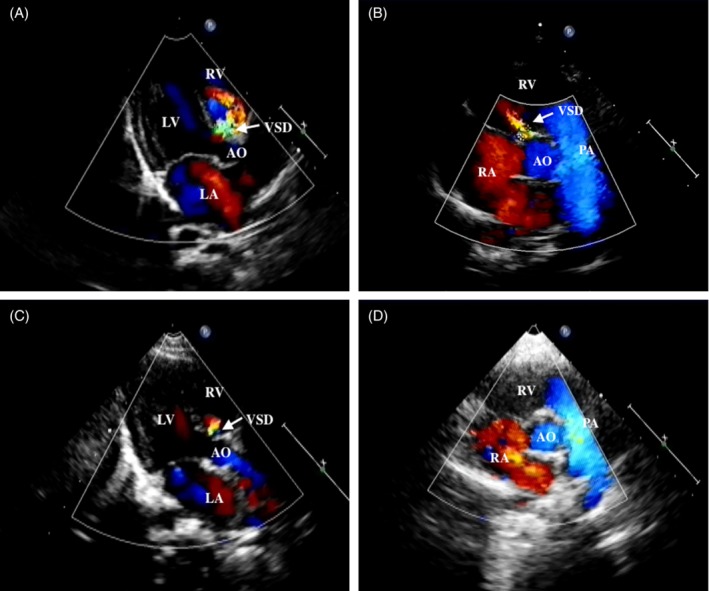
Show a process of ventricular septal defect (VSD) children spontaneously closed by echocardiography. A case of a 32‐days girl baby who underwent echocardiography due to heart murmur. A, Color Doppler echocardiography shows parasternal long‐axis viewed of a perimembranous defect, and the width of defect was 4.0 mm. B, When she was 90 days, color doppler echocardiography showed the defect of the parasternal short‐axis had been reduced, and the width of defect was 3.1 mm. C, When she was 8 months, this defect became smaller to 2.0 mm. D, When she was 14 months, the defect by echocardiography has closed spontaneously, and heart murmur also disappeared. AO, aortic; LA, left atrium; LV, left ventricular; PA, pulmonary artery; RA, right atrium; RV, right ventricular; VSD, ventricular septal defect
